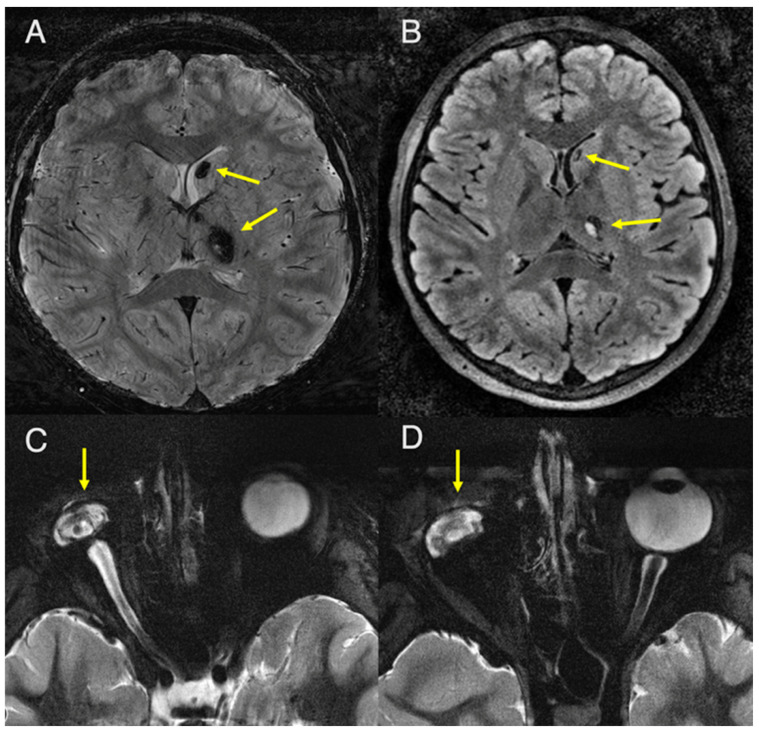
Axial 7T MRI slices obtained from the patient’s younger brother with Coats disease. (A) T2* image (TR/TE/Slice thickness: 1560 ms/20 ms/1.5 mm) and (B) 3D-FLAIR-SPACE image (TR/TE/Slice thickness: 7000 ms/396 ms/0.4 mm) of the whole brain show coarse basal ganglia calcifications, predominantly involving the thalami and left caudate nucleus (arrows). (C,D) Axial 7T T2 STIR images of the orbits (TR/TE/TI/Slice thickness: 6000 ms/67 ms/320 ms/2 mm) in subsequent axial slices show right microphthalmia, retinal detachment, intraocular proteinaceous debris, and intraocular blood products, representing chronic sequelae of Coats disease (arrows).
